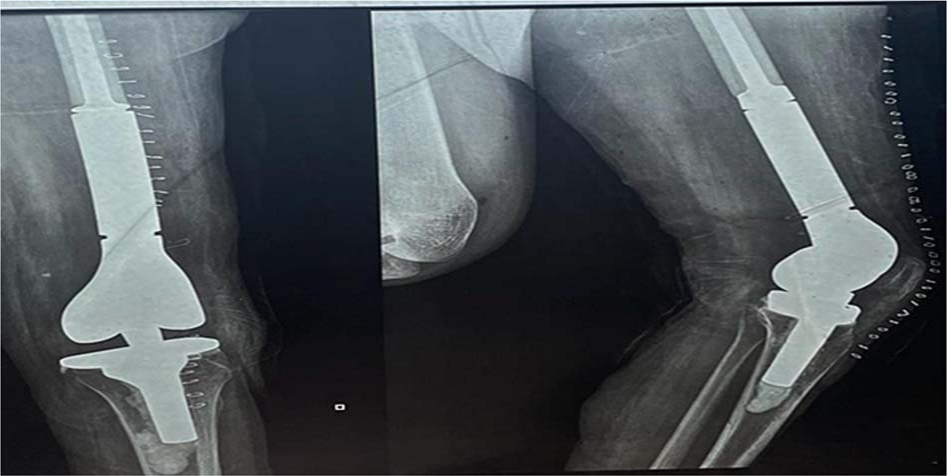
The X-ray after surgery showed a mega prosthesis with good implant alignment.
